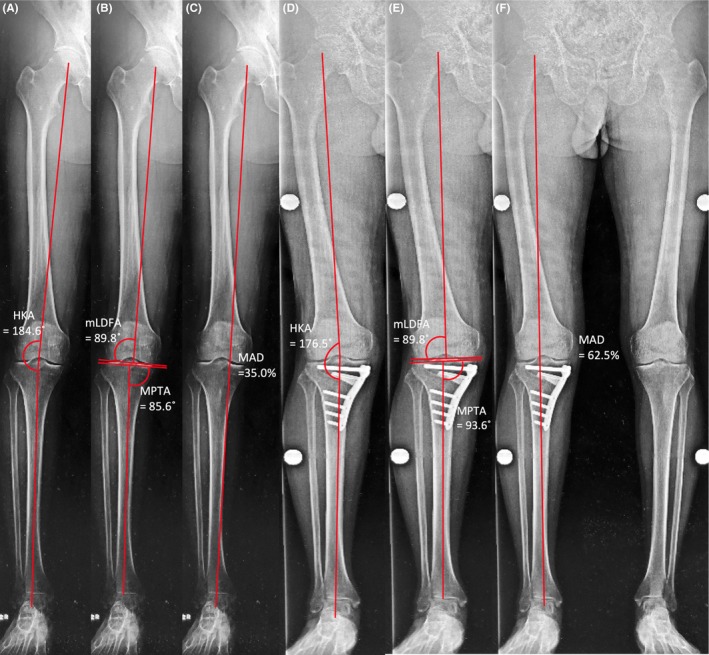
Preoperative (A, B, C) and postoperative (D, E, F) whole‐leg standing radiographs. The hip‐knee‐ankle angle (HKA) was corrected from varus 4.6° (A) to valgus 3.5° (D). The preoperative mechanical lateral distal femoral angle (mLDFA) and medial proximal tibial angle (MPTA) were 89.8° and 85.6°, respectively, and the postoperative mLDFA and MPTA were 89.8° and 93.6°, respectively (B, E). The mechanical axis deviation (MAD) was 35.0% from the medial edge of the tibial plateau preoperatively (C) and 62.5% postoperatively (F)
